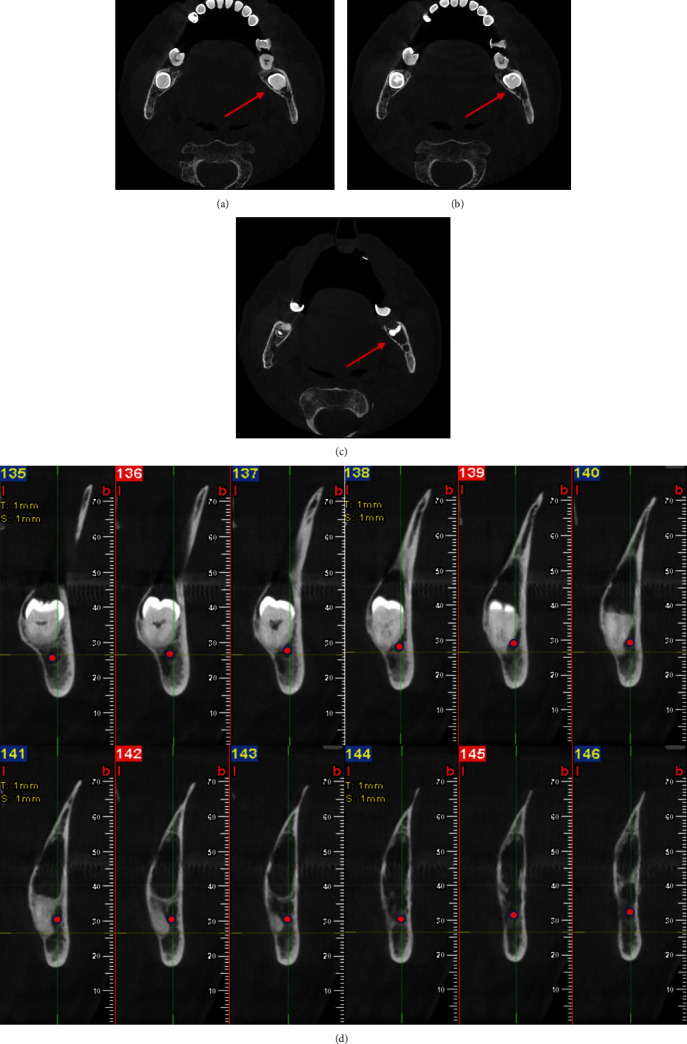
(a–c) 3D dimensions and boundaries of the follicular cyst (red arrow through CBCT axial section and (d) relationship with inferior alveolar nerve (IAN), located buccally (redpoint) through cross-sectional imaging.
